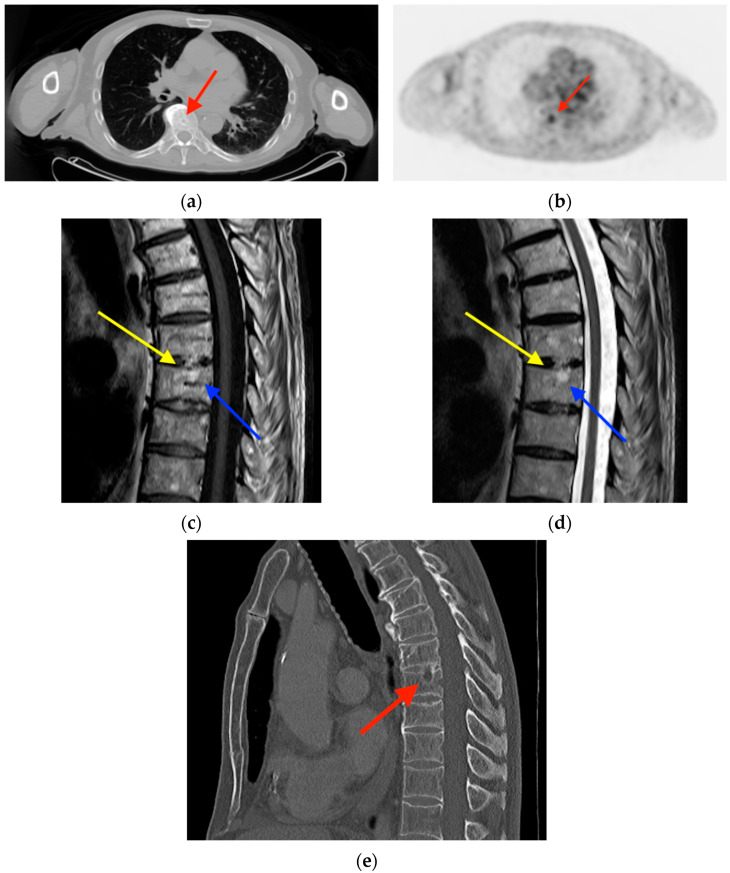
Initial CT (a) and FDG PET images (b) obtained in a 72-year-old man with head and neck squamous cell carcinoma demonstrated a lucent lesion in the T8 vertebral body corresponding with a subtle area of focal radiotracer uptake (red arrows). Sagittal T1-weighted (c) and T2-weighted (d) MRI images obtained three days after the FDG PET/CT show the bony defect at the superior end plate of T8 (yellow arrows) with intraosseous herniation of the intravertebral disk (blue arrows). (e) CT obtained 37 months after the initial FDG PET/CT shows a persistent lesion (red arrow), in contrast to acute calcific discitis, in which the lesion typically resolves. CT = computed tomography. FDG PET/CT = fluorine-18 fluorodeoxyglucose positron emission tomography/computed tomography.
